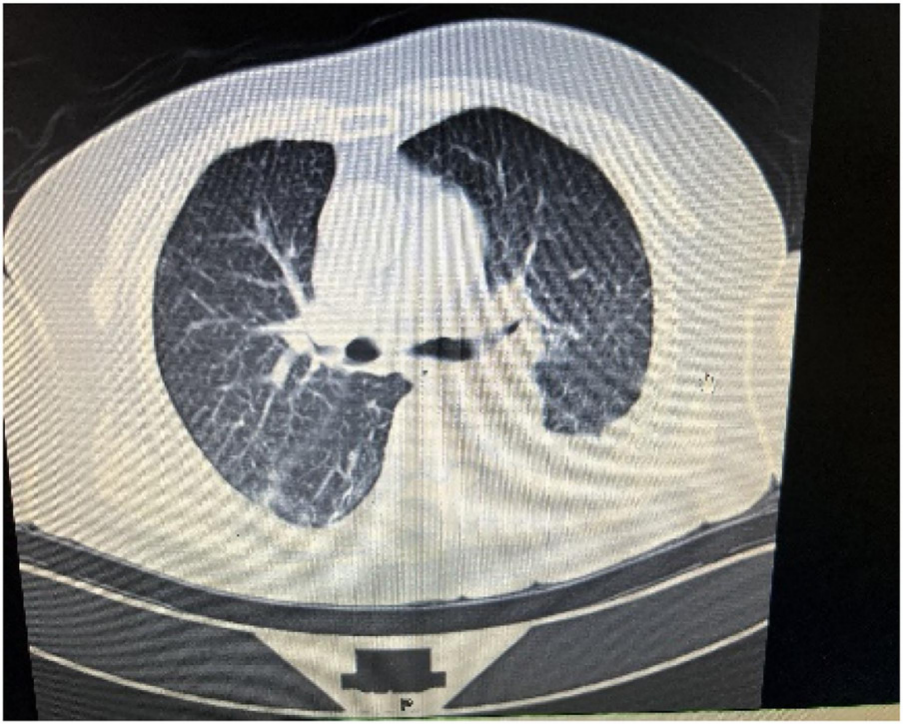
Chest CT on admission. Extensive inflammatory changes were observed in both lungs, accompanied by a marked left-sided pleural effusion.
